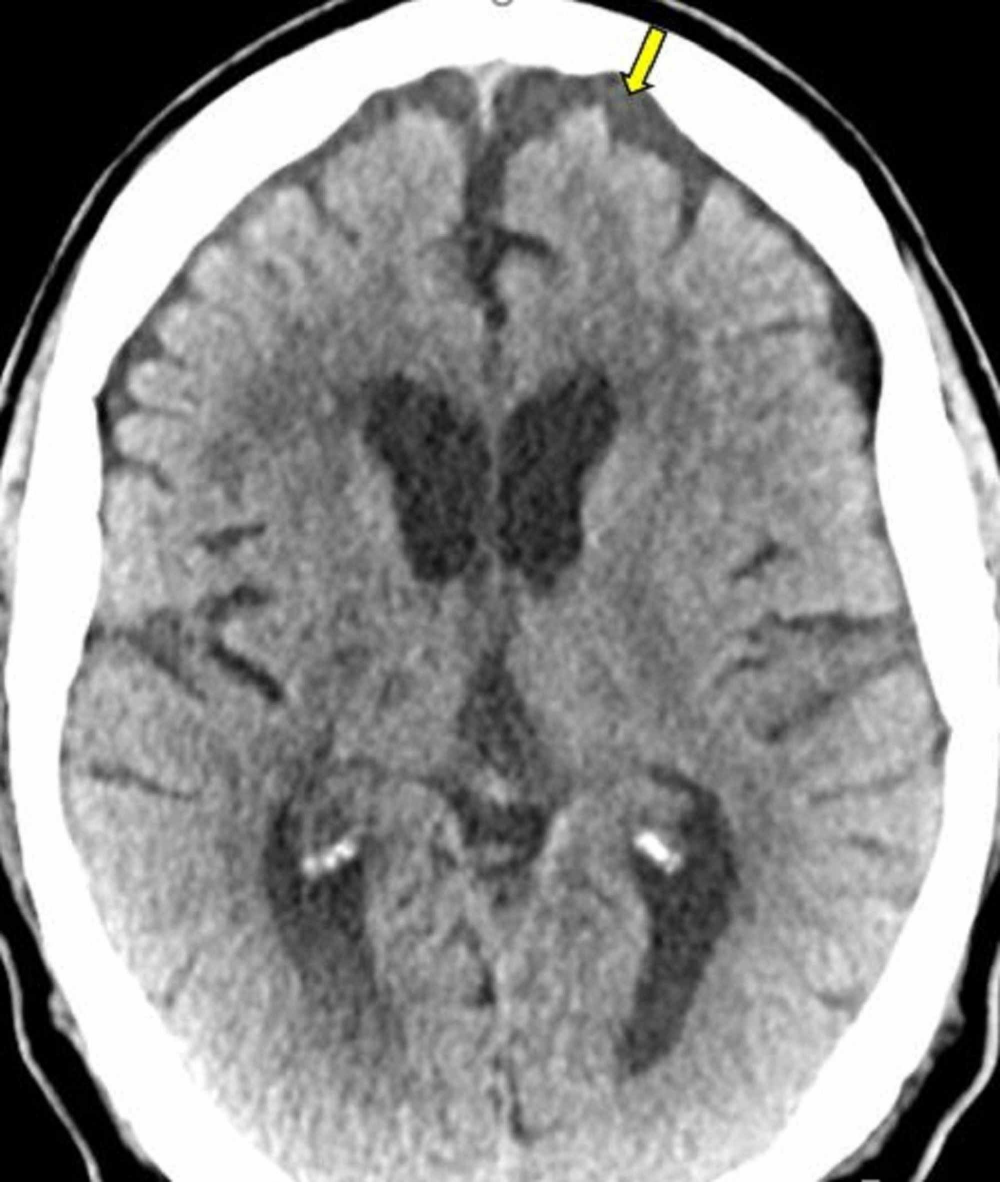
CT of the head showing age appropriate involutional changes (yellow arrows). No acute intracranial process.
